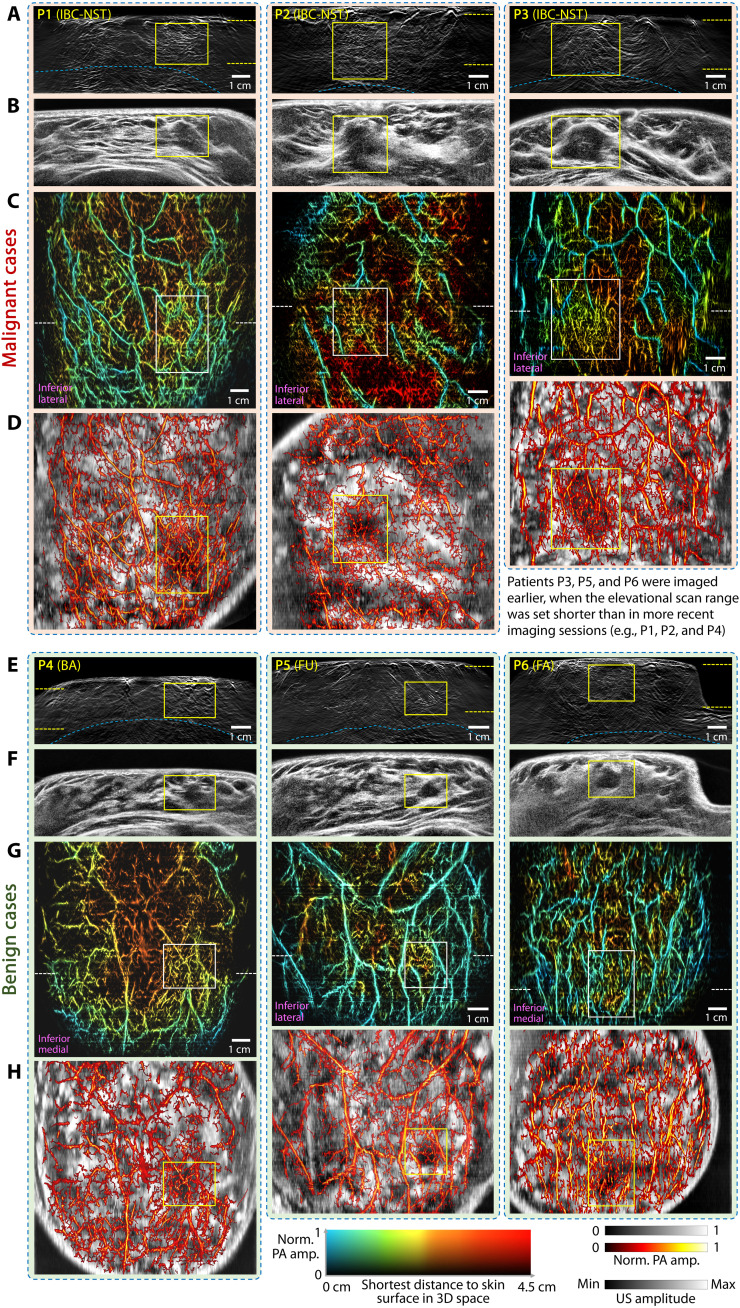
HDMI of patients with breast tumors. (A and E) PACT images of tumor-containing slices on the transverse plane, with tumor-affected regions labeled by yellow boxes and the chest walls outlined by blue dashed lines. Images are cropped either near the chest wall or at a depth of 5 cm. (B and F) URCT of the same cross sections as shown in (A) and (E), demonstrating concordance in tumor locations. (C and G) PACT images of breast cross sections [marked by yellow dashed lines in (A) and (E)] on the coronal plane, with tumors enclosed by white boxes. The white dashed lines indicate the position of the transverse cross sections shown in (A) and (E) and (B) and (F). (D and H) Fused images of PACT and URCT on the coronal plane, with PACT vascular features displayed in a hot color map overlaid on the grayscale ultrasound anatomy. The tumor-affected regions are outlined with yellow boxes. BA, breast adenosis; FA, fibroadenoma; FU, maintained BI-RADS 3 during a 6-month follow-up; IBC-NST, invasive breast carcinoma of no special type.
